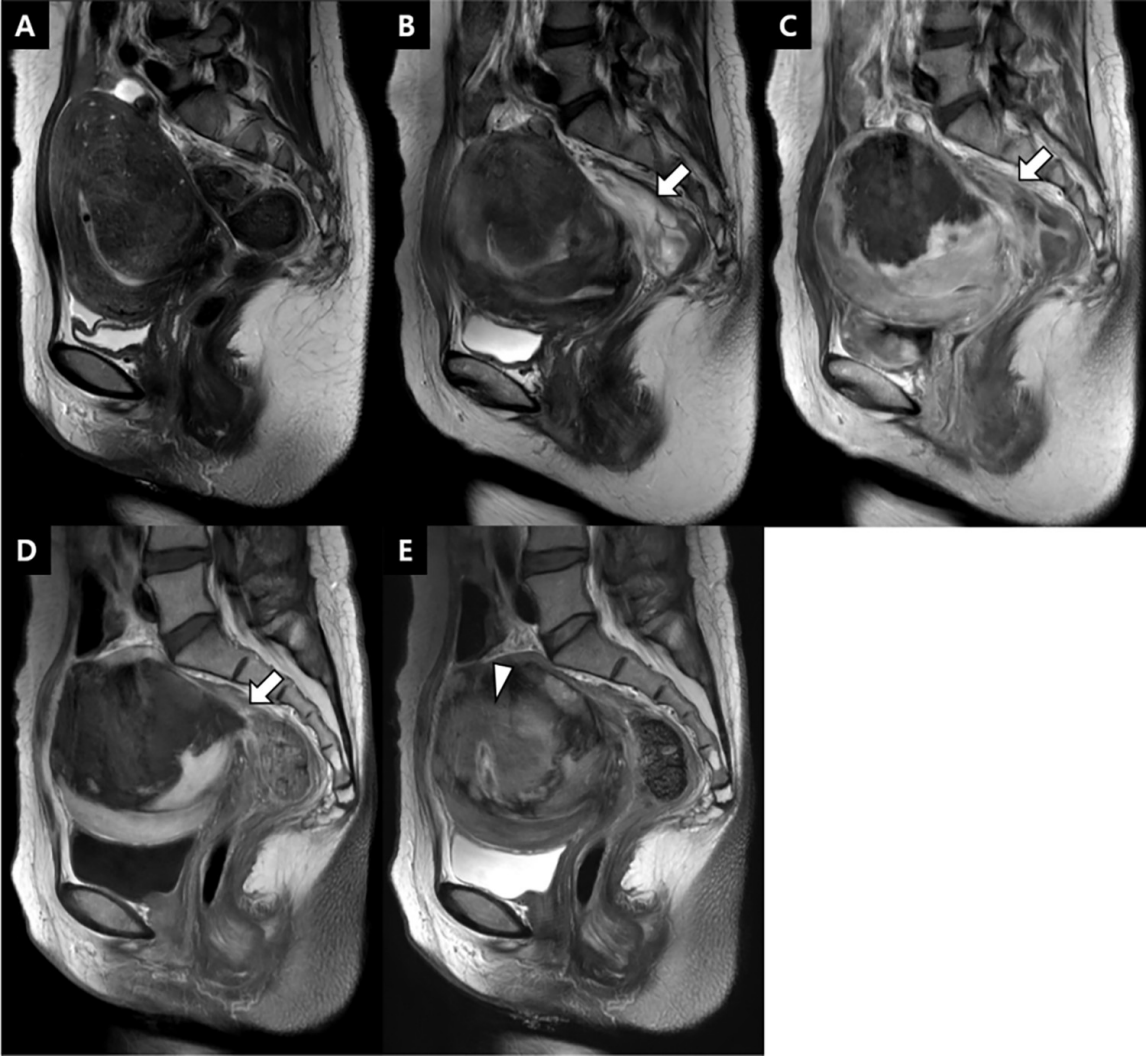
An adverse event after high-intensity focused ultrasound treatment. Sagittal T2-weighted images (A, B, E) and contrast-enhanced T1-weighted images (C, D) of a 46-year-old woman with adenomyosis (arrowhead). (A) Before HIFU treatment. (B, C) Increased signal intensity and enhancement along the anterior wall of the upper rectum adjacent to the adenomyosis was detected in the immediate posttreatment images (arrows). Her symptoms improved after conservative management, and she was discharged. (D, E) At the 1-month follow-up, the signal intensity of the rectal wall was normalized (arrow). Note the shrinkage of the treated adenomyosis (arrowhead).
